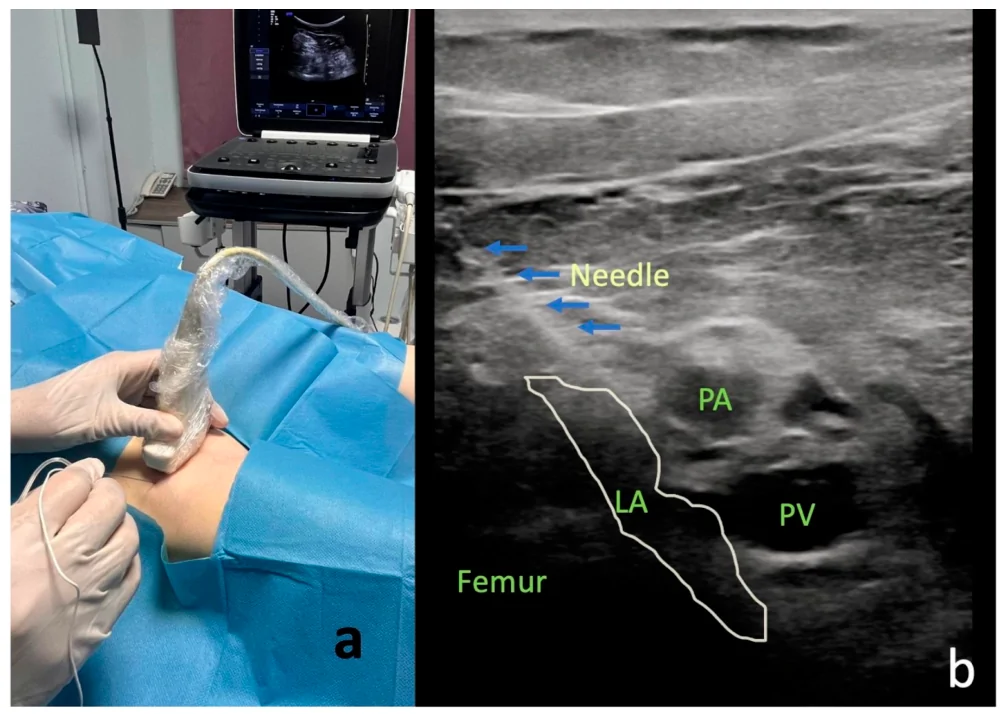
(a) Position of the ultrasound probe and needle during the IPACK block. The patient is in the prone position. The operator stands lateral to the patient with the ultrasound machine positioned in front of the operator. (b) Ultrasound image showing the needle trajectory, local anesthetic distribution, and relevant anatomical structures during the IPACK block. PA: popliteal artery, PV: popliteal vein, LA: local anesthetic.
